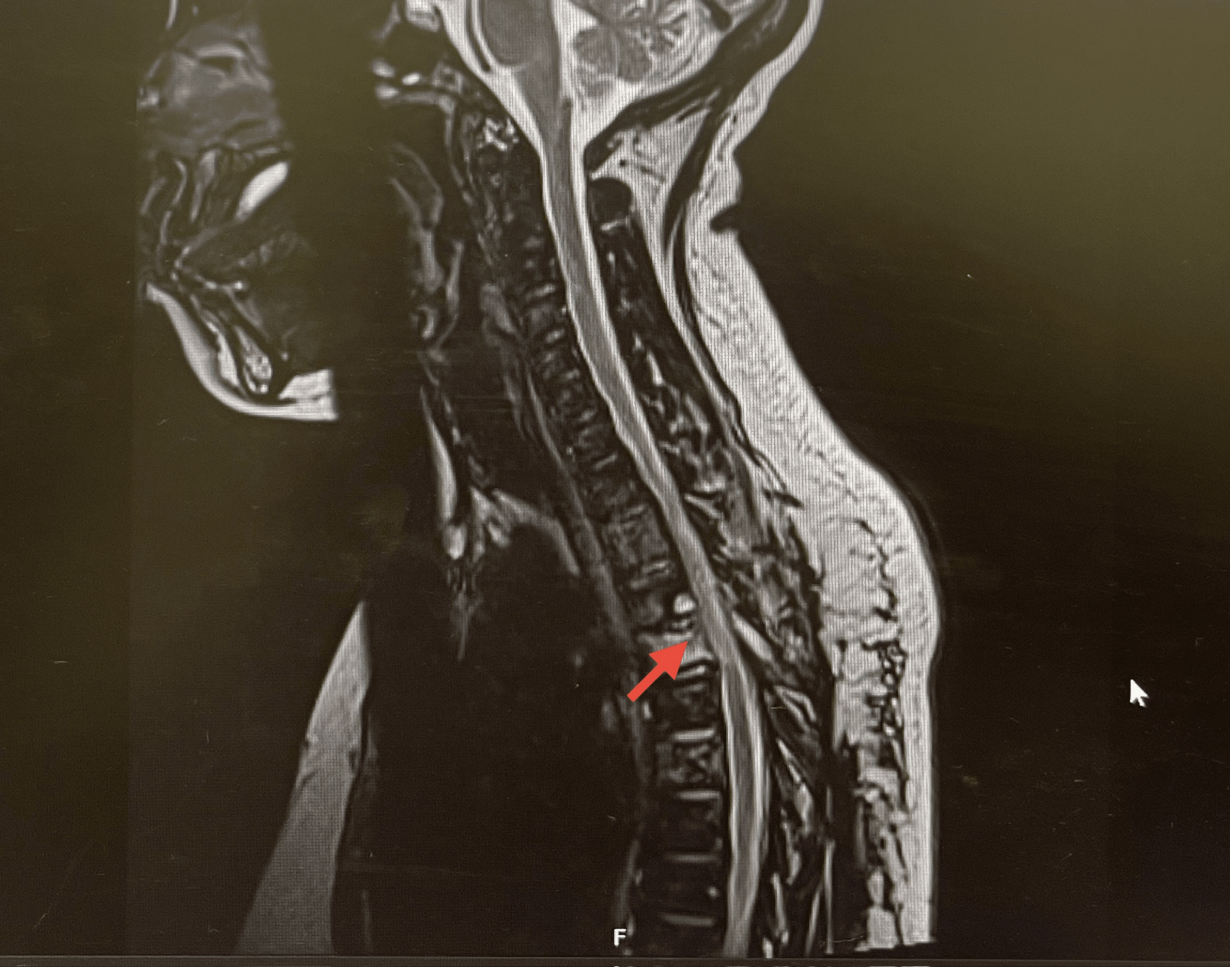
Follow-up MRI demonstrating T4-T5 hyperintense signal (arrow) consistent with radiation-induced myelitis.
